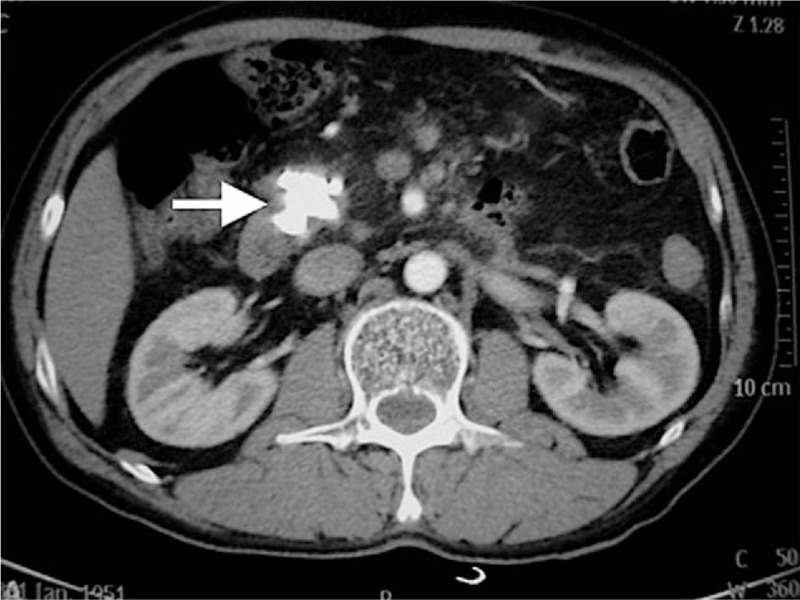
Abdominal computed tomography scan demonstrating a large high-density shadow located at the head of the pancreas (white arrow).
